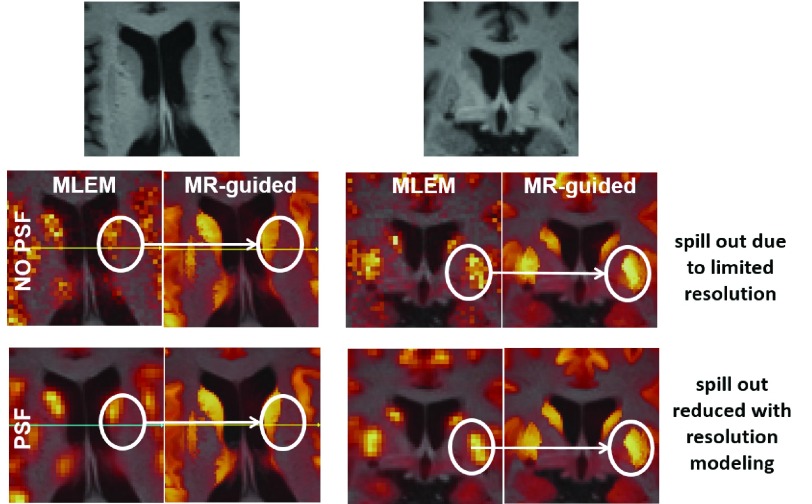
Impact of resolution modeling in MR-guided reconstructions. In the top row, a patch on a transverse (left) and coronal (right) slice of the T1-weighted image. In the middle row, the same slices for the MLEM and MR-guided reconstructions without resolution modeling. In the bottom row, images reconstructed with resolution modeling.
